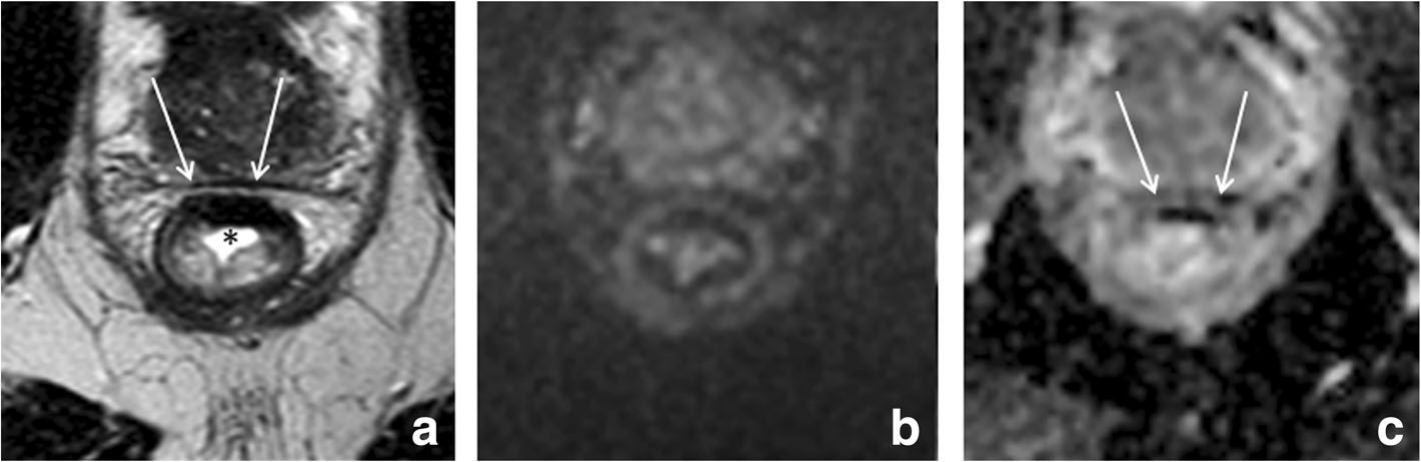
Pitfall of low ADC in fibrosis. Example of the post-CRT T2-weighted MRI (a), b1000 diffusion-weighted MRI (b), and ADC map (c) of a male patient treated for a low rectal tumor. The patient was a complete responder. A fibrotic wall thickening is present in the anterior rectal wall (arrows). On the ADC map (c), a markedly low signal is observed in the fibrosis, which is due to the high collagen content in fibrosis (that has a short T2 relaxation time) and should not be mistaken for restricted diffusion due to tumor. On DWI (b), the signal in the rectal wall is homogeneously low, indicating that there is no restricted diffusion. Note again the star-shaped shine-through of high T2 signal (*) caused by some fluid in the rectal lumen
